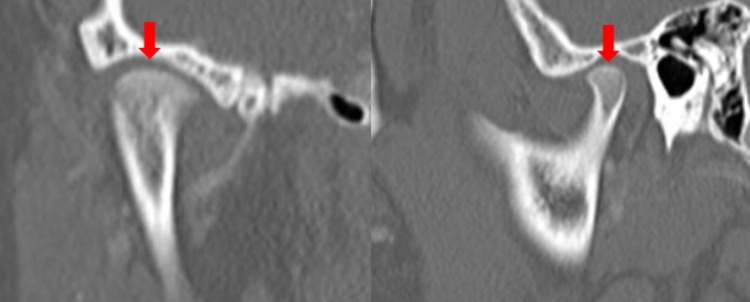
CT images of normal right TMJ shown in (A) coronal view and (b) sagittal view. Normal curved articulation (arrows).
